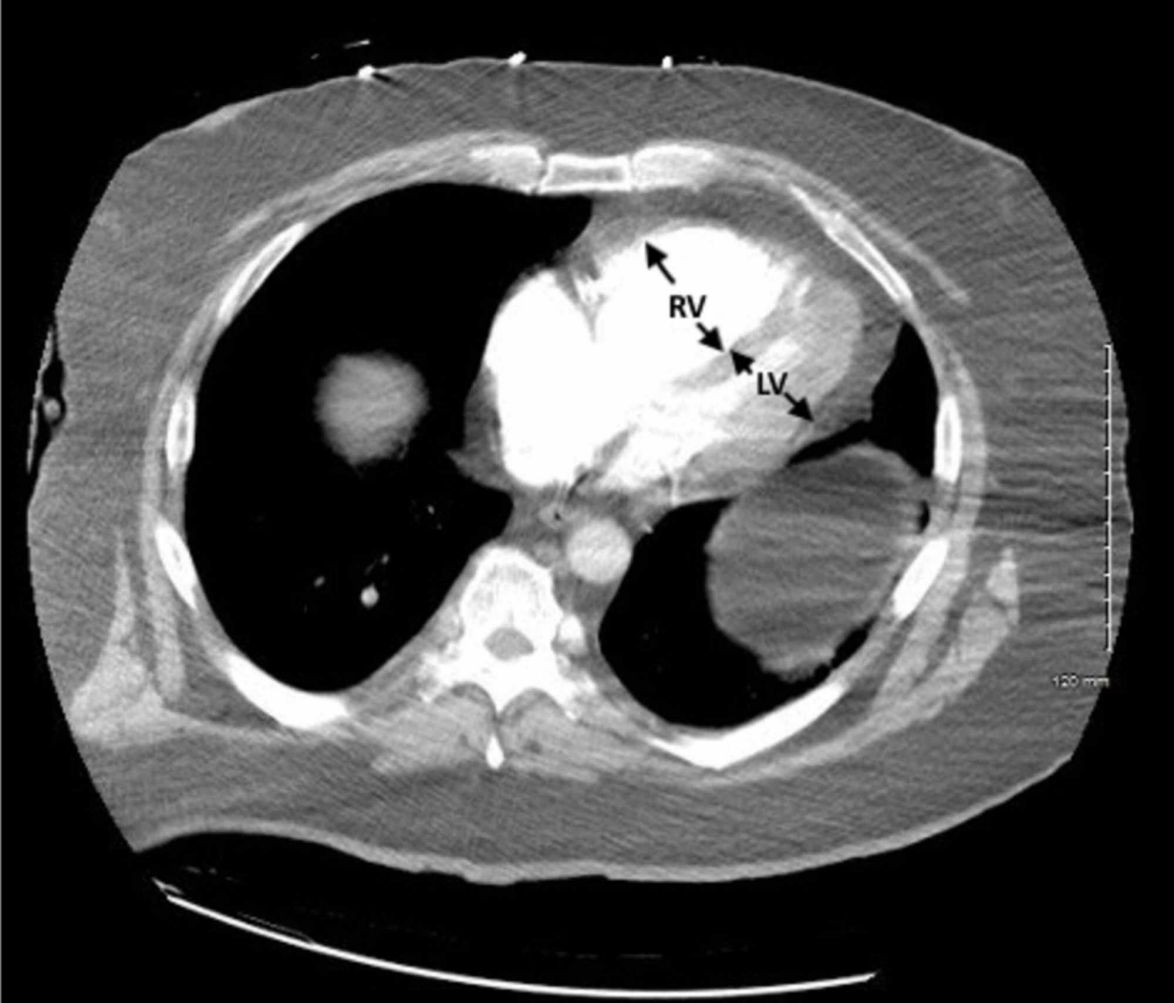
CTPA demonstrating evidence of right heart strain with RV dilatation (arrows). CTPA, computed tomography pulmonary angiogram; LV, left ventricle; RV, right ventricle.
